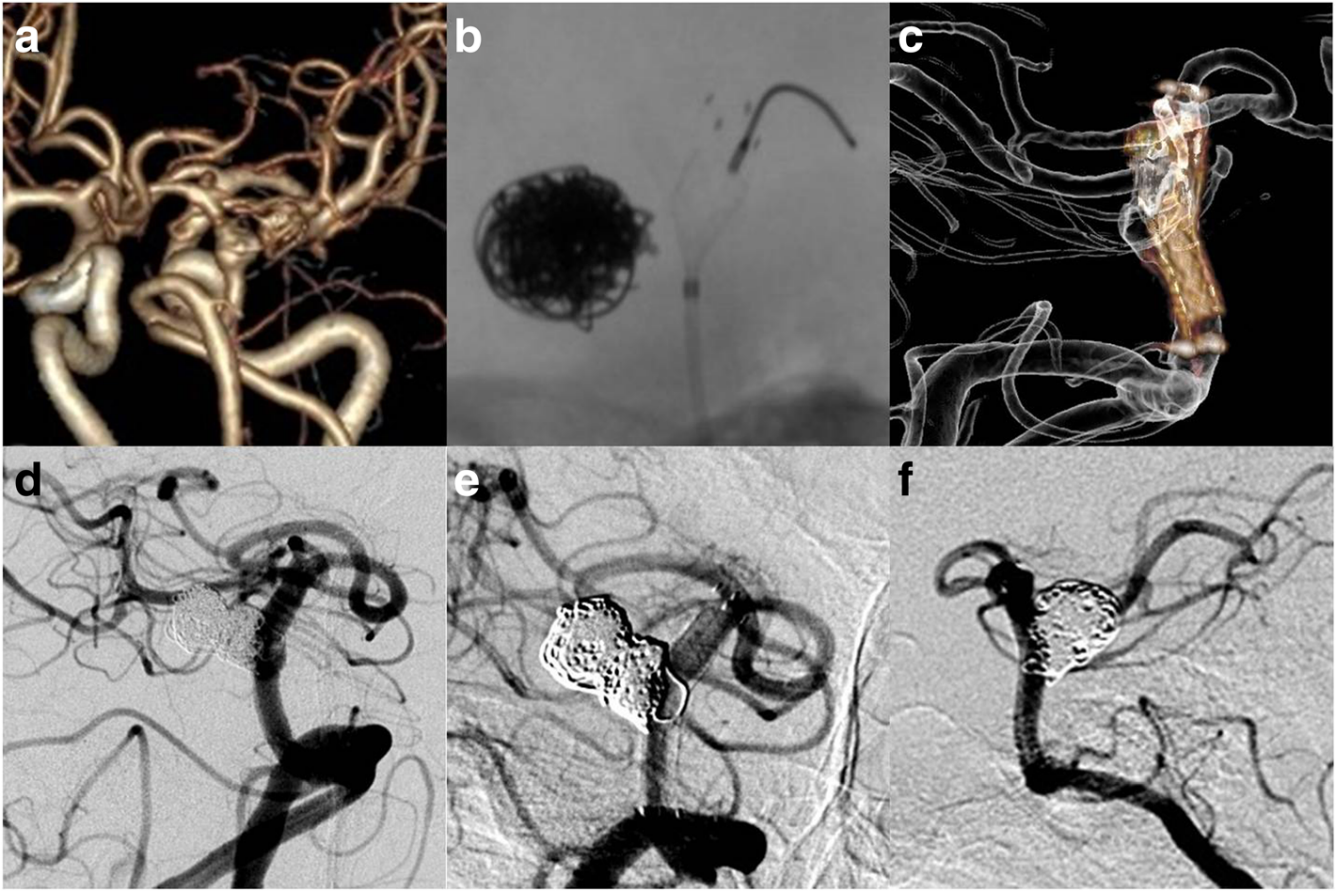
a CTA on admission suggested the recurrence of the aneurysm with fusiform morphology. b The distal end of the PED was deployed proximal to the distal end of the previous Enterprise stent. c Dyna-CT indicated that the entire PED was deployed within the Enterprise. d Adjunctive coiling was performed to secure the aneurysm. Right anterior oblique (e) and lateral view (f) of DSA indicated complete occlusion of the aneurysm on follow-up 6 months later
